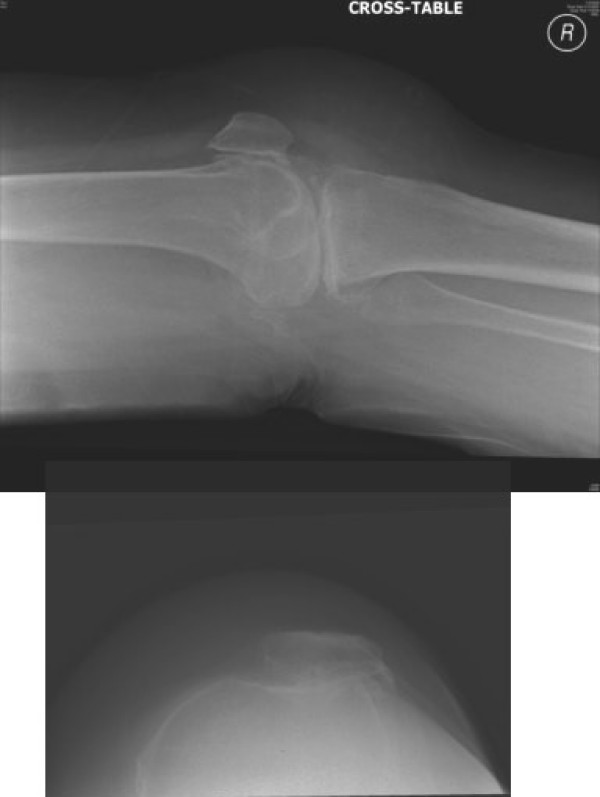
Preoperative lateral x-ray of the right knee and skyline of the patella. Showing severe arthritis and maltracking of the right patella.
